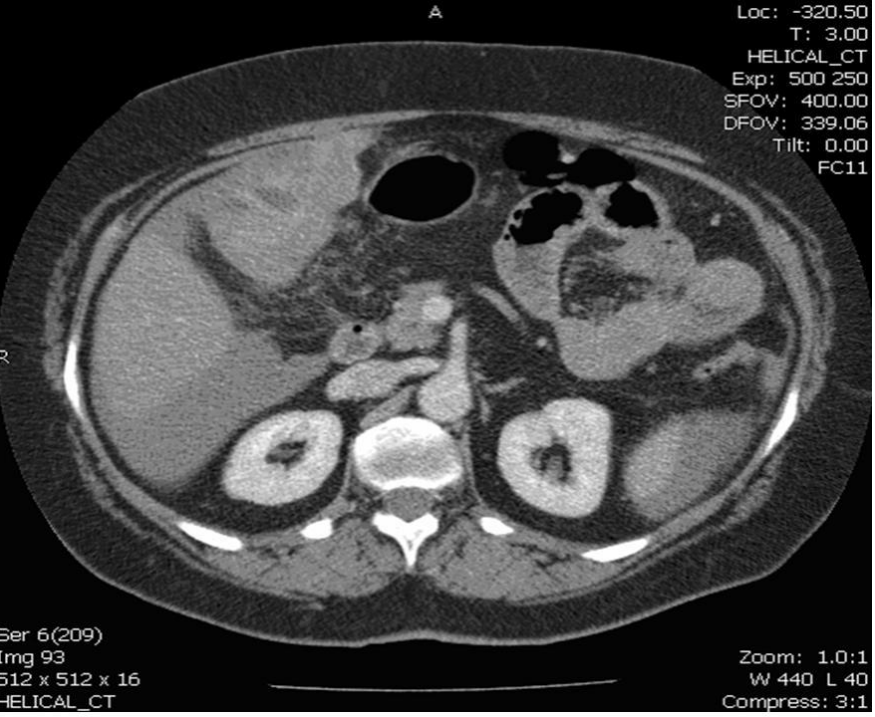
CT scan picture of the GIST close to liver and distinctly separate from gastric lumen.
